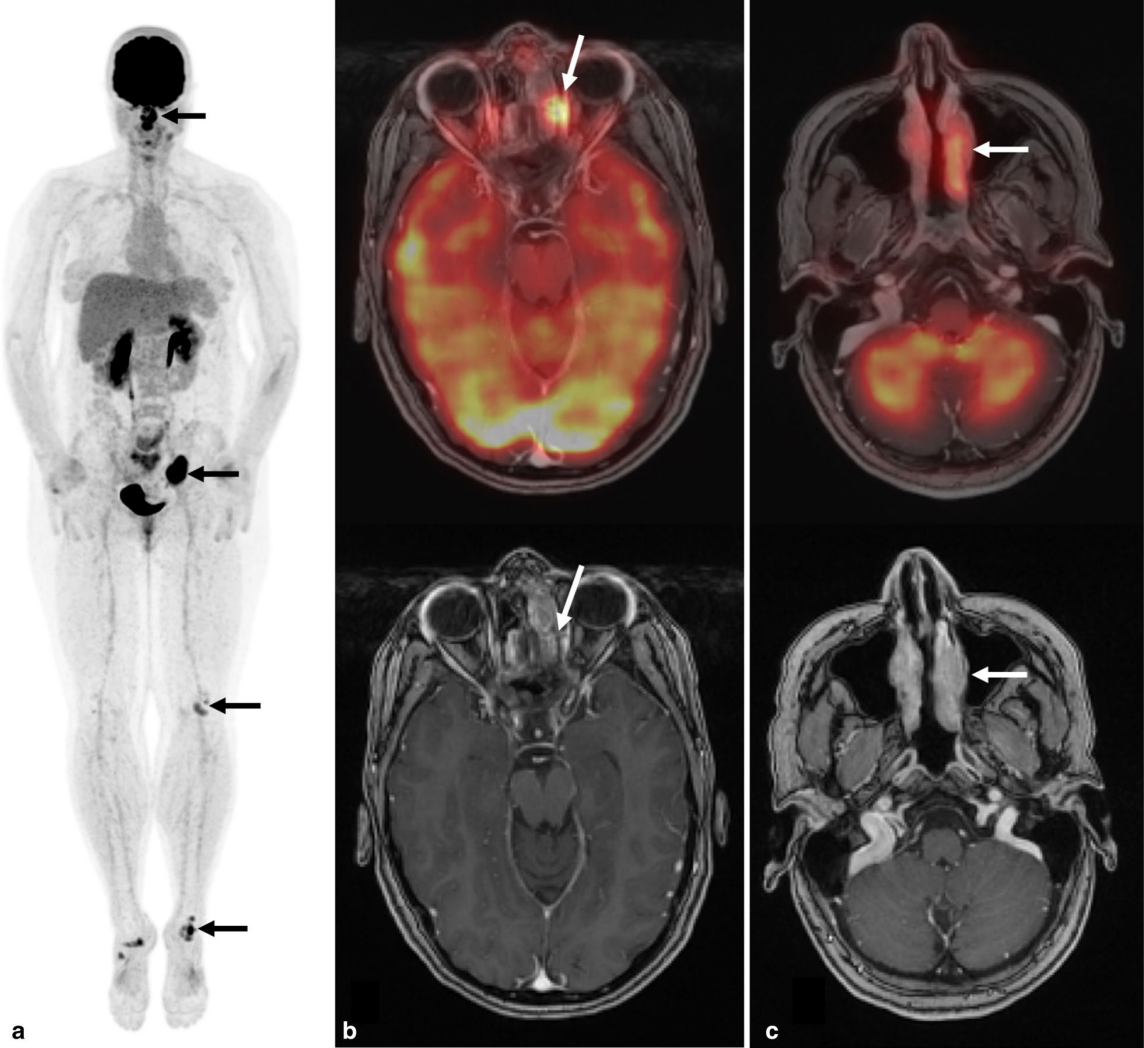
Whole-body ^18^F-FDG PET co-registered with contrast-enhanced maxillofacial MRI demonstrates sinus lesions. a Whole-body FDG PET maximum intensity projection image demonstrates intense uptake in the nasal cavity, paranasal sinuses, left pelvis, left knee and left ankle (black arrows). Nonspecific mild uptake in the right ankle, and left side of the neck are also seen. b Mildly enhancing infiltrative lesion in the left anterior ethmoidal sinus in the Gadolinium-enhanced maxillofacial MRI, demonstrates intense FDG uptake in the fused PET/MRI axial image (SUV_max_ 10.6, white arrow). c Enhancing infiltrative mass in the left middle nasal turbinate, in the Gadolinium-enhanced maxillofacial MRI, demonstrates intense FDG uptake in the fused PET/MRI axial image (SUV_max_ 7.7, white arrow)
